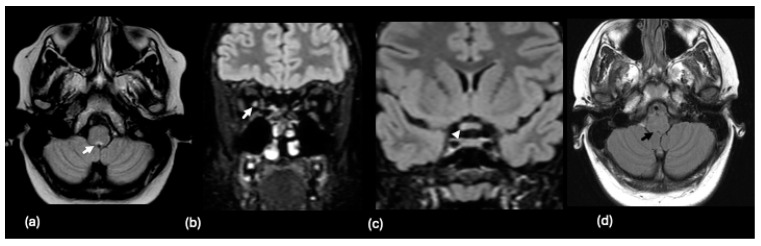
A 10-year-old male presenting with left lower limb weakness, pain, and hyperreflexia. (a) Axial FLAIR image demonstrates a non-enhancing, small, focal hyperintense signal abnormality along the posterior inferior medulla (white arrow). On coronal FLAIR (b,c), there is asymmetric edema in the right optic nerve (white arrow) and subtle swelling and signal abnormality of the right optic chiasm (white arrowhead). (d) 2-year-follow-up MR shows resolution of medullary signal abnormality (black arrow) on axial FLAIR.
